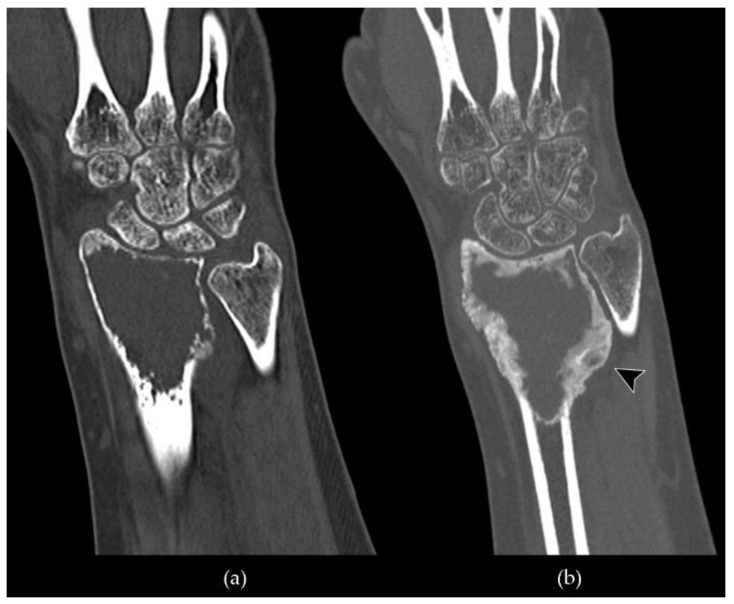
Coronal CT scan of a giant cell tumor of bone of the distal metaepiphysis of the radius treated with denosumab: (a) a pre-treatment image shows an osteolytic lesion with noticeable cortical thinning and endosteal scalloping; (b) a post-treatment image demonstrates peripherical osteosclerosis around the lesion with neocortex formation (arrowhead).
